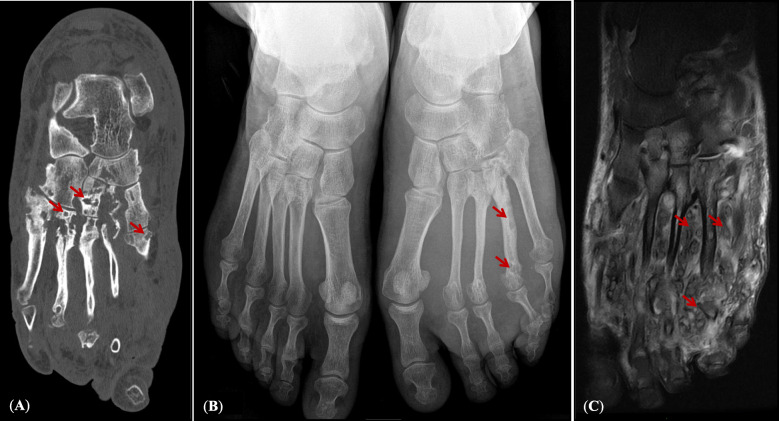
a Left foot tomodensitometry demonstrating metatarsals osteolysis and thickened soft parts; b Foot X-rays with thickened soft parts of the left foot and the 4th metatarsal osteolysis; c Soft tissue infiltration by bacteria and bone involvement of the left foot demonstrated using T2 sequence fat-sat pulses in MRI
